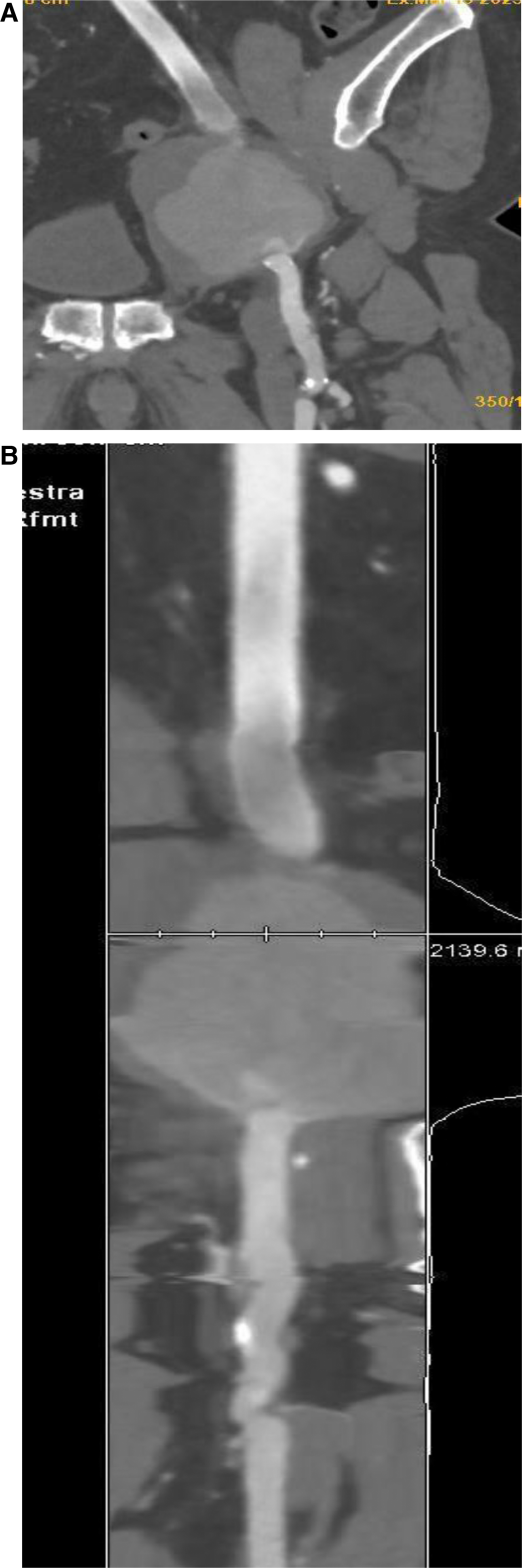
Computed tomography 3D multiplanar reconstruction (A) describes the presence of pseudoaneurysm at the level of the left branch of the bifemoral aorto-by-pass (B) better displayed in the coronal view (B).
